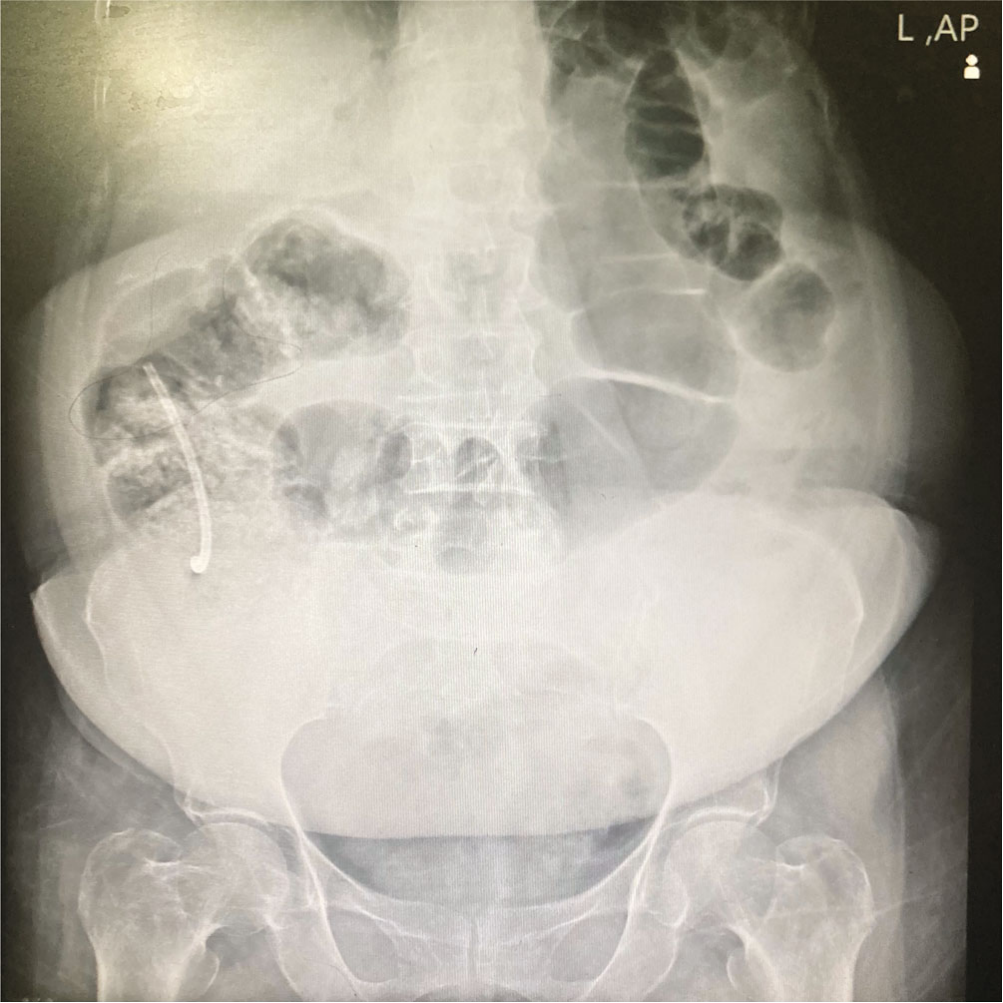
Abdominal radiography showed the stent at the RLQ.
